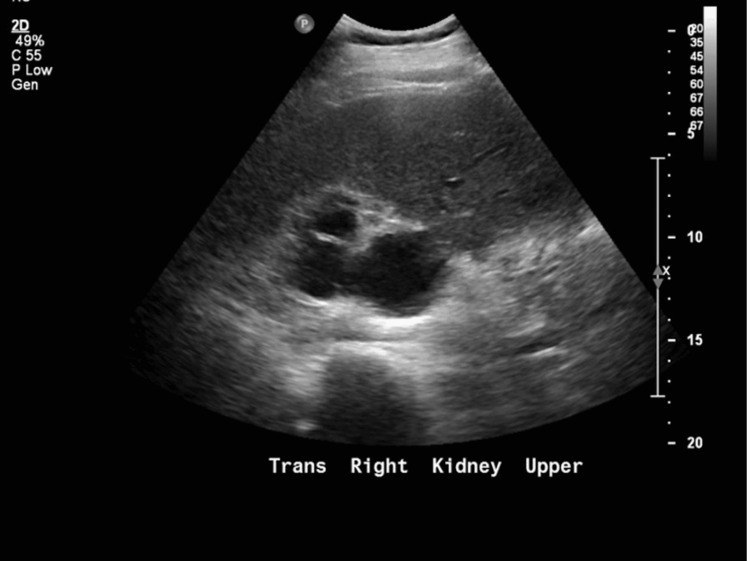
Renal ultrasound showing severe hydronephrosis with moderate cortical thinning and hydroureter.
